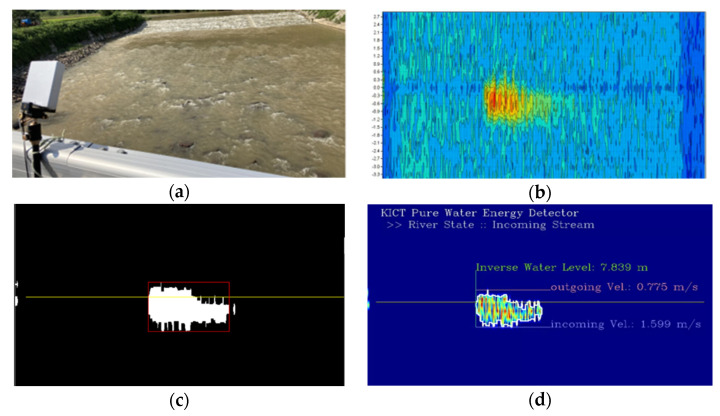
(a) On-site water monitoring utilizing WAVE-Surf; (b) actual range–Doppler map observed from (a); (c) blob-labeling result (red box) and flow energy mask generated from (b) (N = 3); and (d) calculated water level and velocity from (c).
